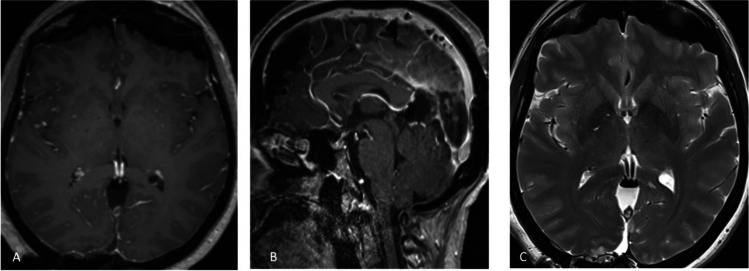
Post-operative T1-contrast enhanced MRI (A) axial and (B) sagittal images, showed complete resection with patent deep venous system and straight sinus. T2-weighted MRI did not reveal any complications such as occipital lobe edema (C)
